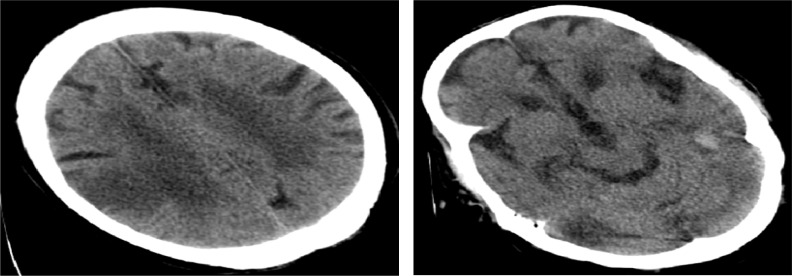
Brain CT scan after 24 hours with asymptomatic ICH
